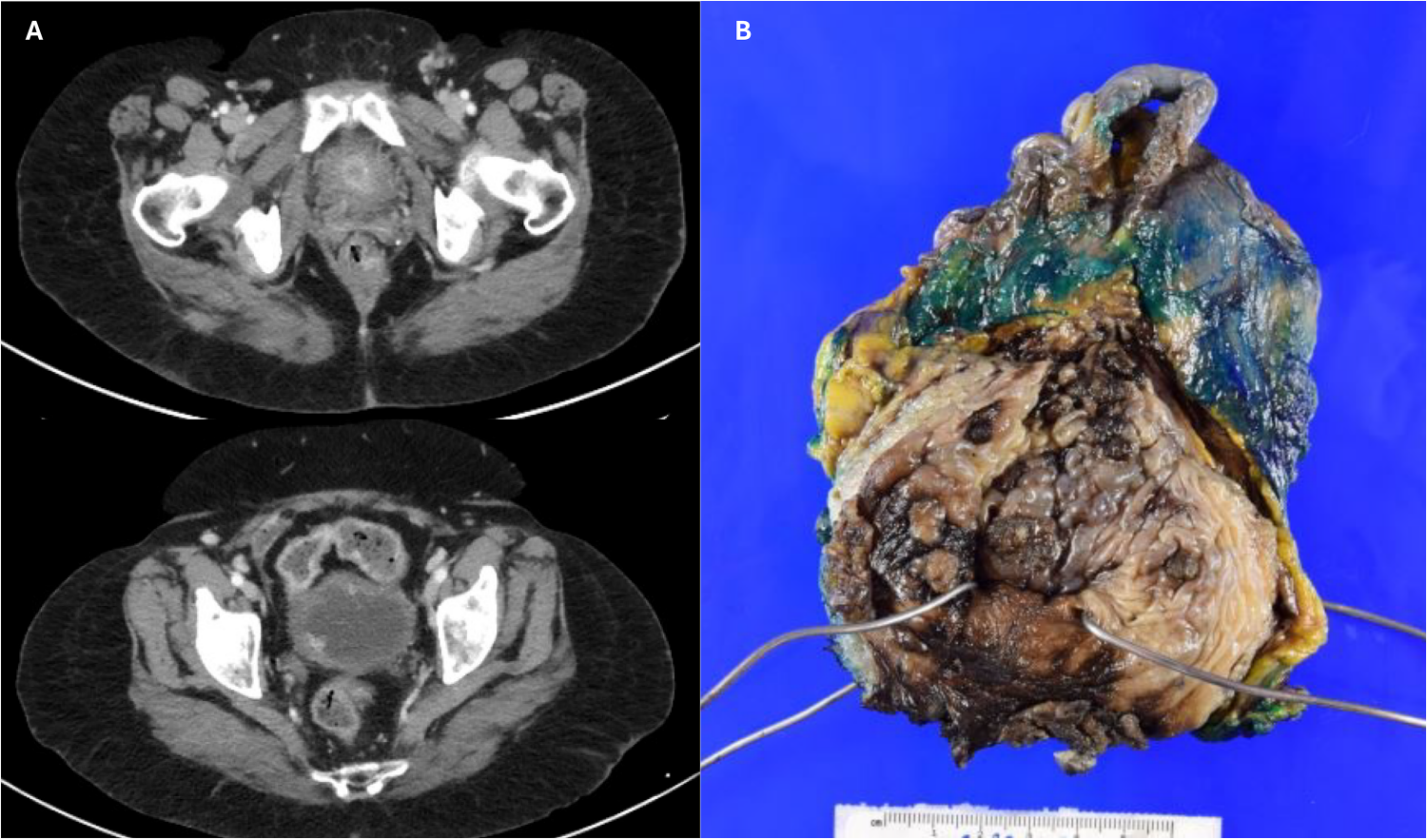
Radiographic and macroscopic findings. (A) PET/CT showing multifocal hyperenhancing lesions in the bladder, the largest at the anterior bladder neck (upper panel) and an additional lesion near the right posterior bladder base (lower panel), (B) gross image of urinary bladder with multiple foci of brown–gray, friable, nodular masses.
